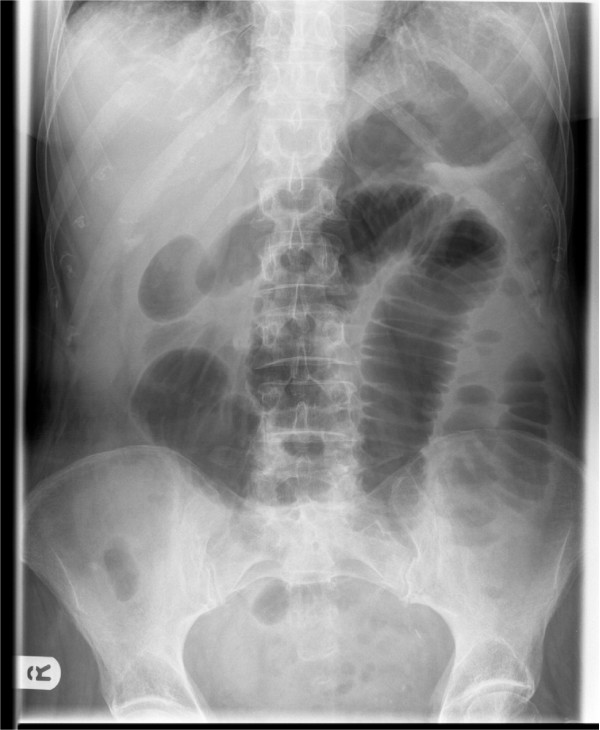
Abdominal X-ray of patient 2 showing evidence of small bowel obstruction.
